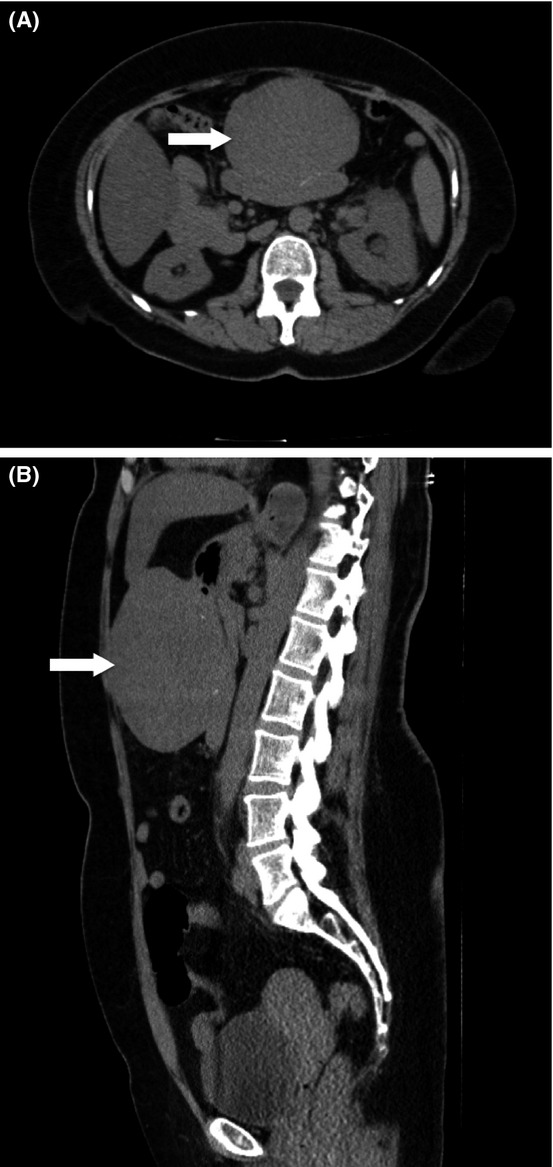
CT scan of patient's abdomen shows an incidentally discovered homogeneous, solid mass that displaced the stomach posteriorly.
